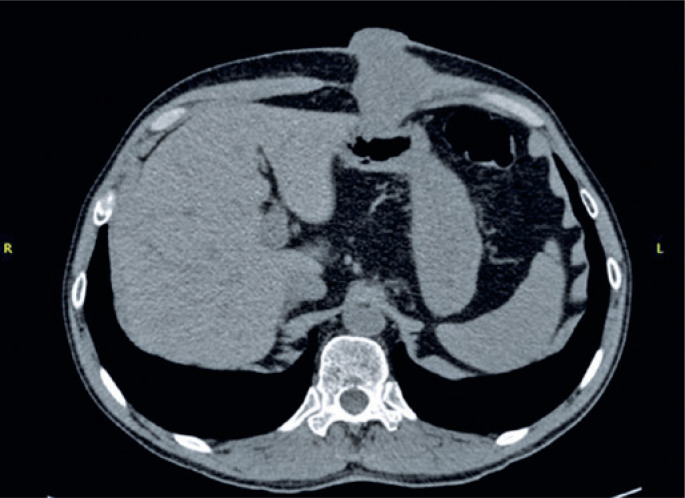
Non-contrast computed tomography, showing postoperative status of previous gastrostomy incision. Irregular contours with dense soft tissues adjacent to the surgical scar, with a component that externalizes, and is in intimate contact with the gastric wall, in the great curvature of gastric body, measuring approximately 56x55x52mm along its longest axes
